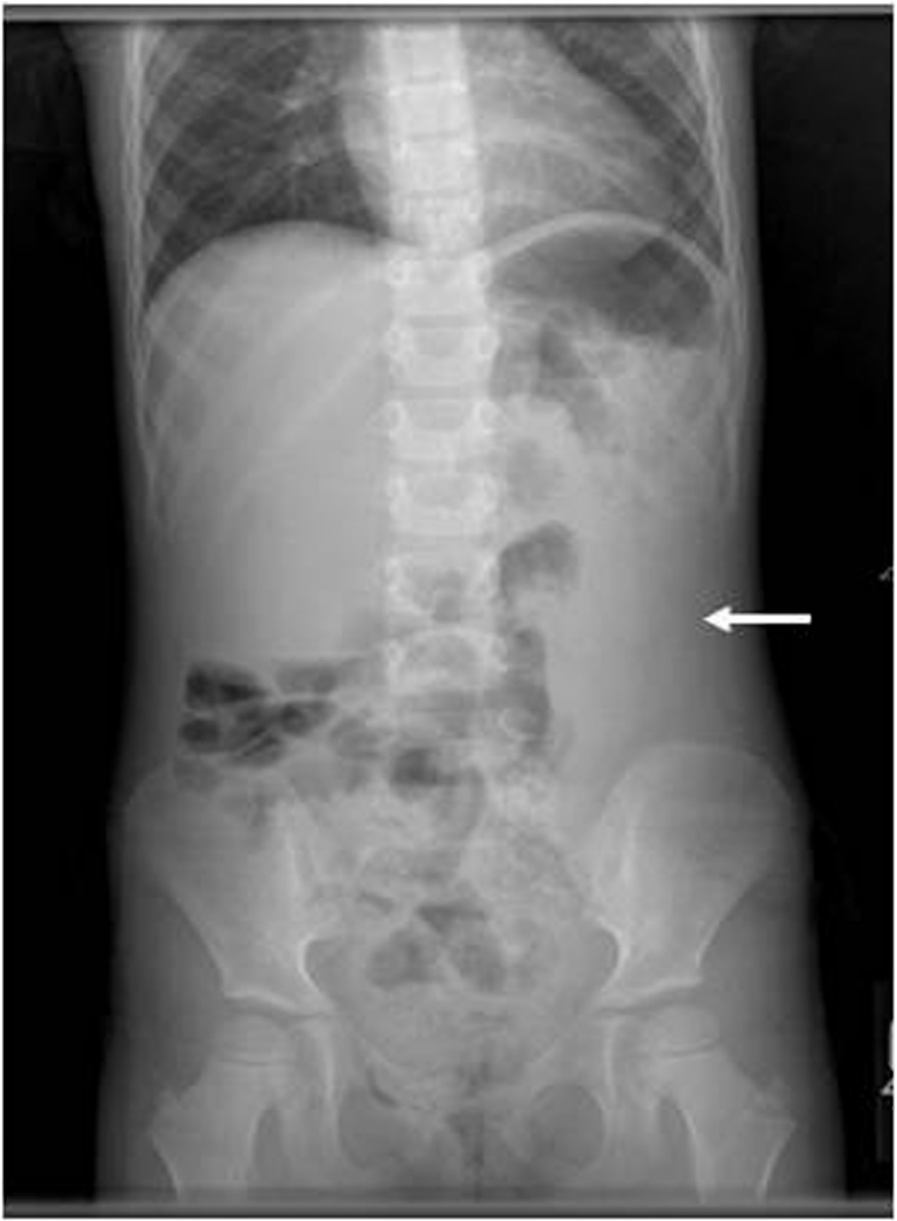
Plain radiograph of abdomen: mass effect noted in left mid-abdomen.
